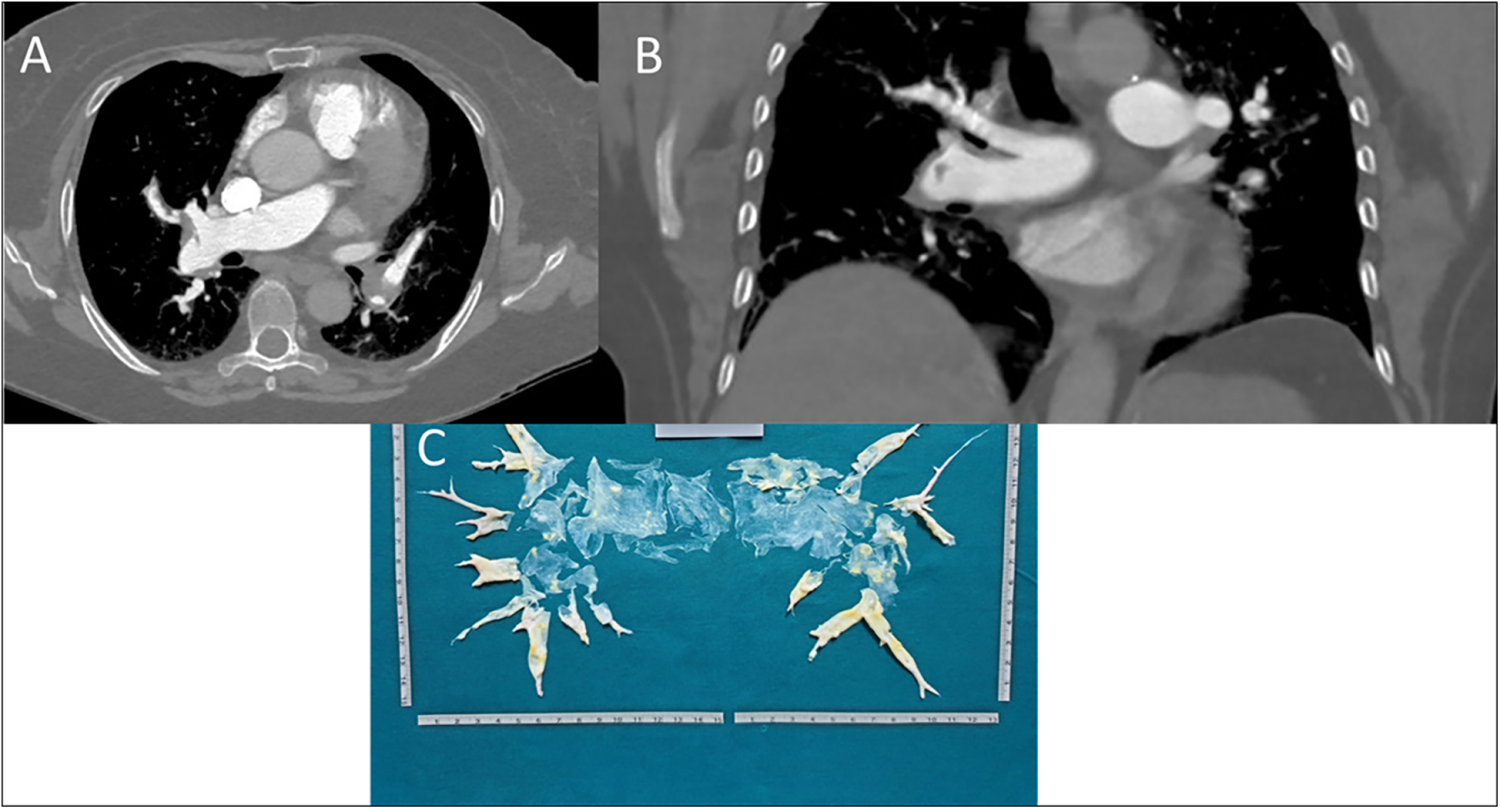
(A) axial scansion of chest CT with contrast. A filling defect of a thrombo-embolic nature in the right pulmonary artery, between the middle and lower lobe tributary branches, can be observed. The arrow also shows small thrombi in the left pulmonary artery branches. (B) Coronal scansion of chest CT with contrast. A filling defect of a thrombo-embolic nature in the branch of the right pulmonary artery tributary to the lower lobe can be observed. (C) Postoperatively surgical finding of pulmonary thromboendarterectomy.
